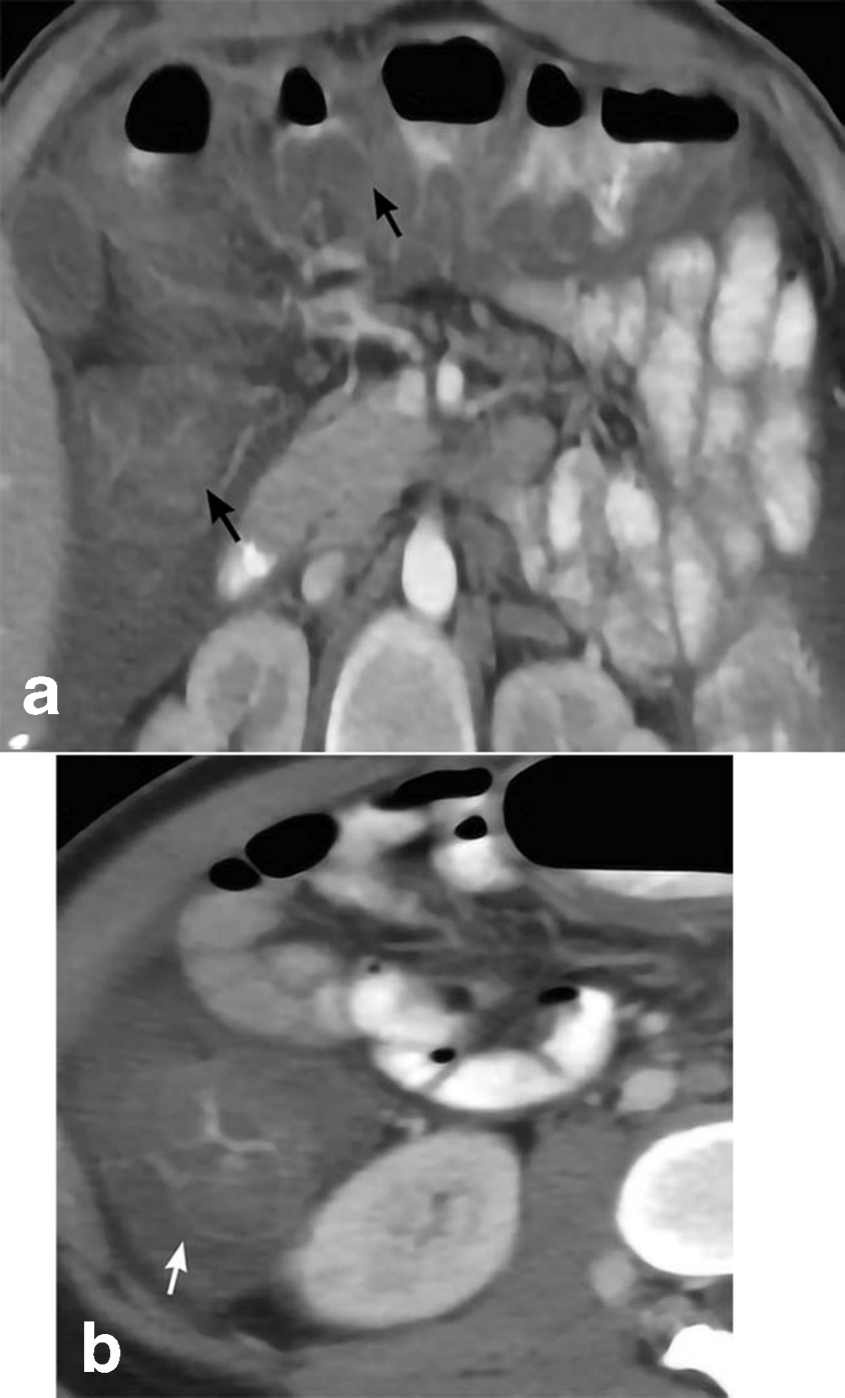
CMV colitis. 23-year-old male with medical history of HIV presents with diffuse watery diarrhea and abdominal pain for 5 days. Axial CT imaging (a) depicts markedly diffuse colonic wall thickening of the ascending and transverse colon (black arrows). The cecum is uniformly thickened (white arrow) as depicted on the axial CT (b). Subsequent sigmoid biopsy reveals colonic mucosa with ischemic changes and numerous viral inclusion cysts compatible with CMV infection. CMV, cytomegalovirus.
